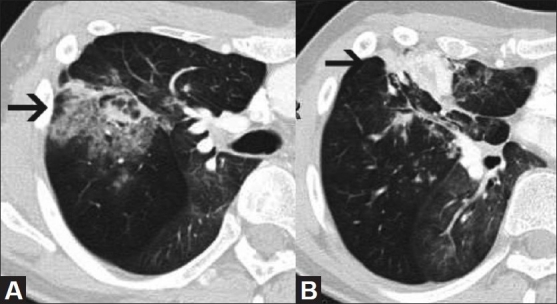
CT scan of the chest at two contiguous levels shows areas of bronchiectasis surrounded by air space opacities (arrow in A) representing hemorrhage within the consolidated right middle lobe, extending to the pleural surface (arrow in B)
